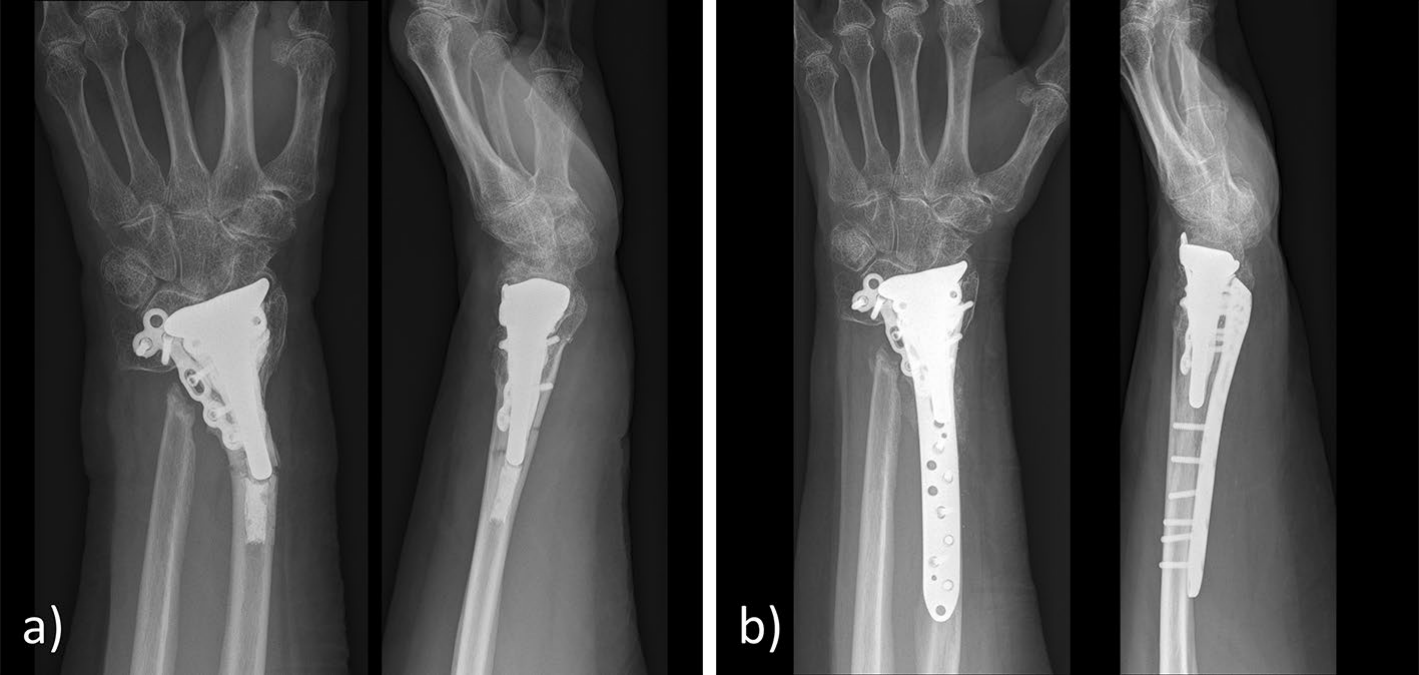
a Radiograph of a periprosthetic fracture after a falling accident. b Radiograph after revision by removing the cement from the proximal fragment, implantation of an allograft and osteosyntheses with a palmar plate
